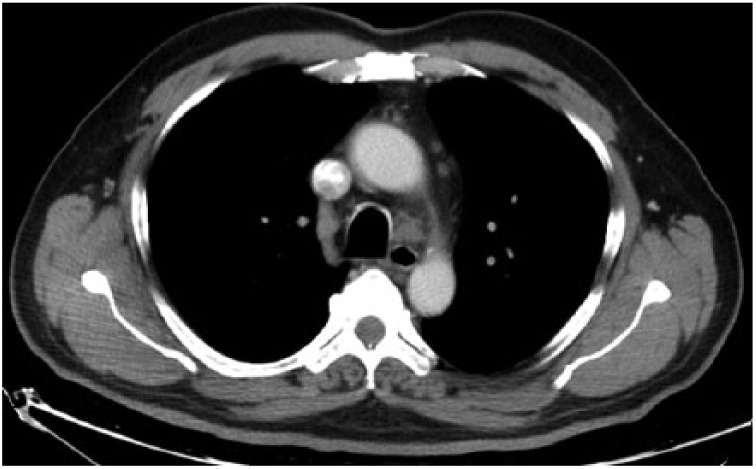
Control thorax HRCT with mediastinal window showing regression in the size of mediastinal lymph nodes.
